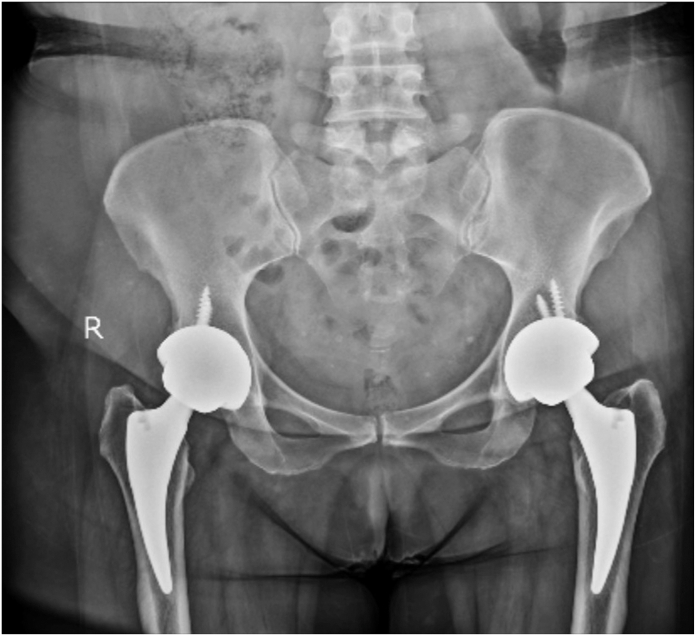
Pelvic anteroposterior X-ray taken at the 12-year follow-up of one of the patients undergoing simultaneous bilateral cementless THA using Continuum cup and Fitmore stem (Zimmer, Biomet).
